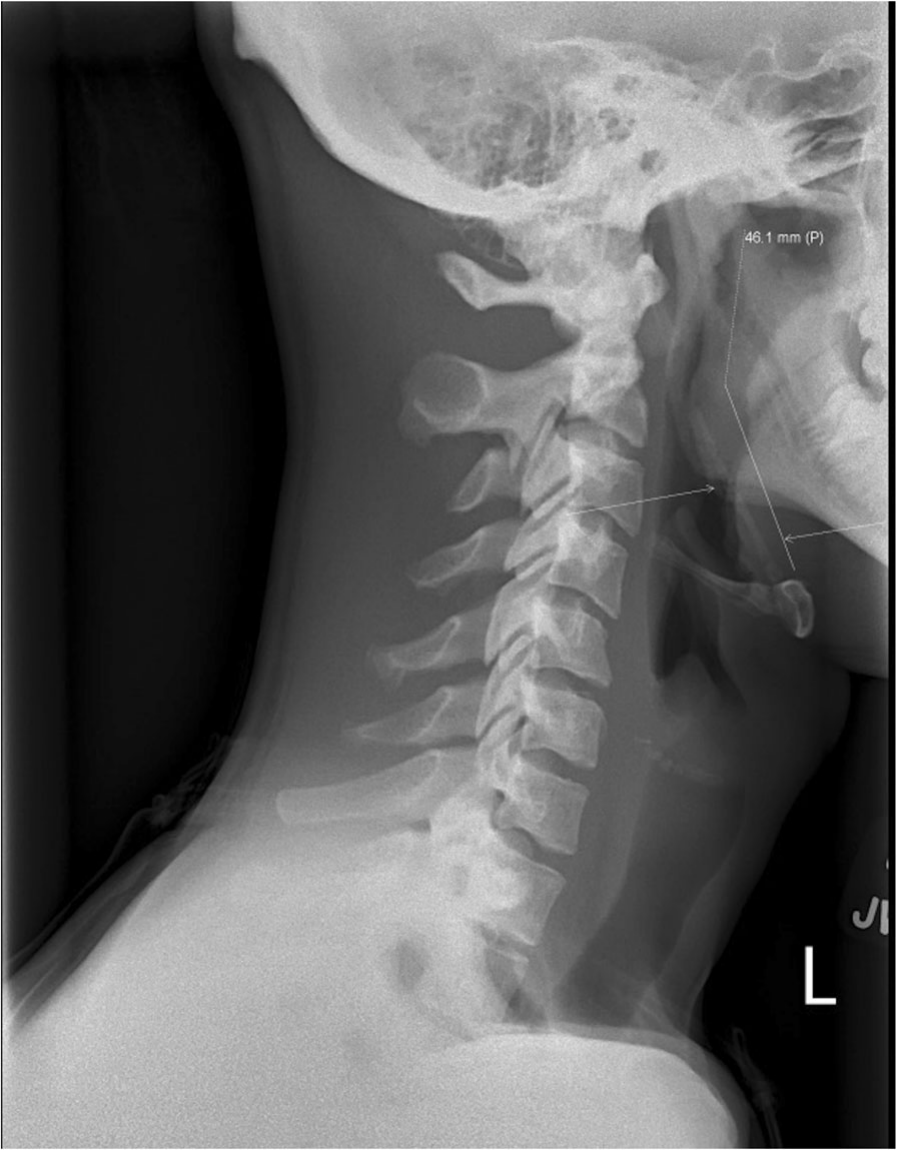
Elongated and calcified stylohyoid ligament on lateral neck radiograph (arrow) measuring 4.6 cm
